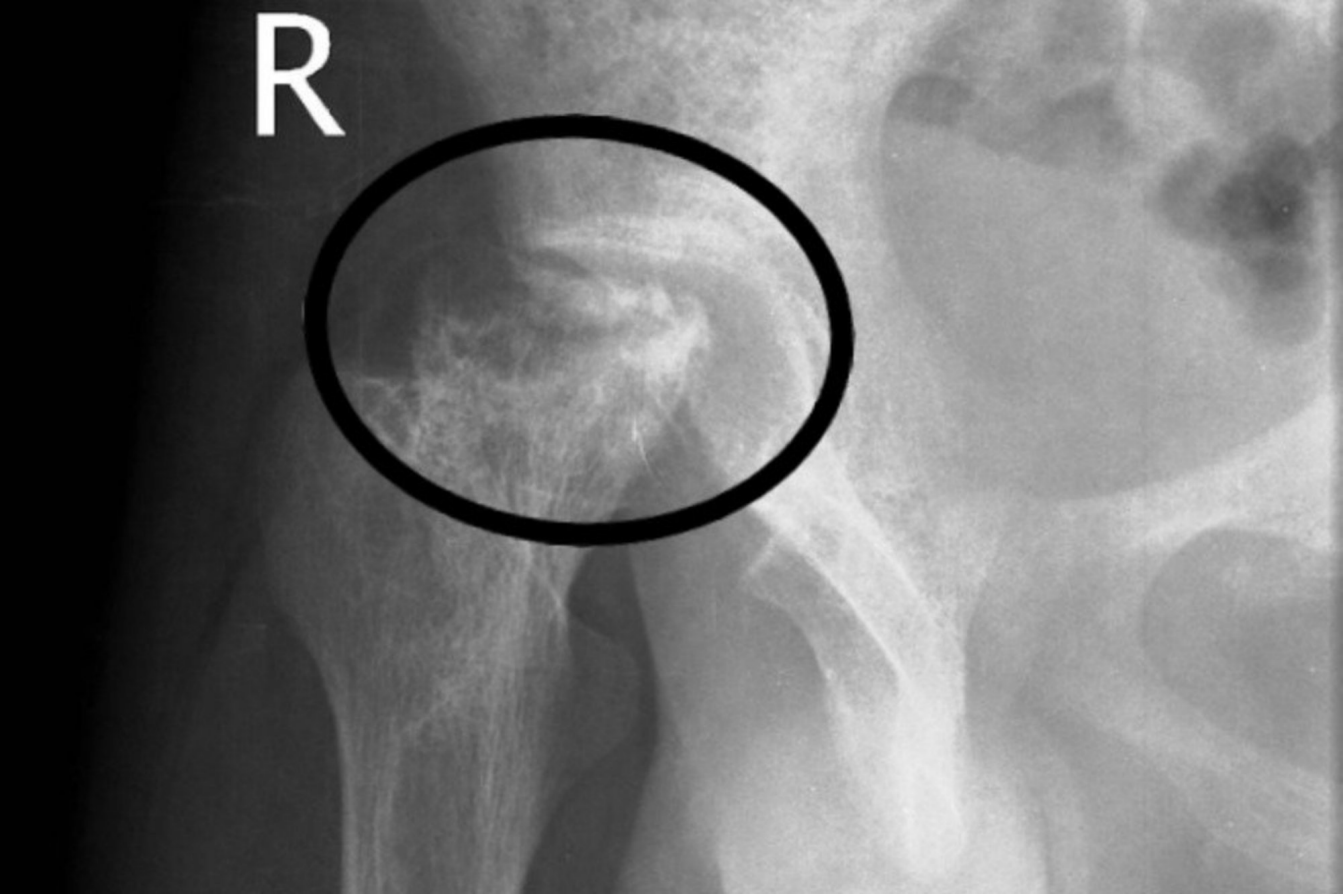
X-ray right hip Advanced osteonecrosis shown in the circle.
